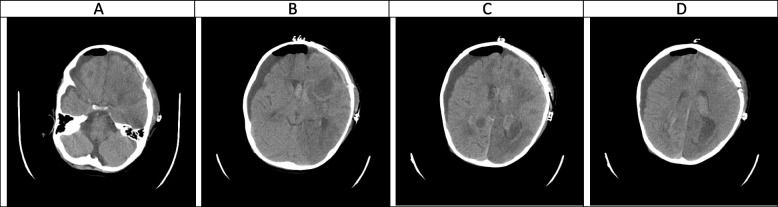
(the brain CT SCAN without contrast, day 9). A-D absent right intraventricular EVD is associated with some air within the intracranial cavity and also subdural fluid accumulation around the right cerebral hemisphere, which is due to a recent operation for EVD removal. No hydrocephalus is seen, however, sign of some amount of intraventricular hemorrhage is seen at present. Mild hemorrhage and edema seen at the site of mentioned large left frontotemporal lesion. A hypodense (abscess) lesions which seen within the cerebral hemispheres again seen in this study
